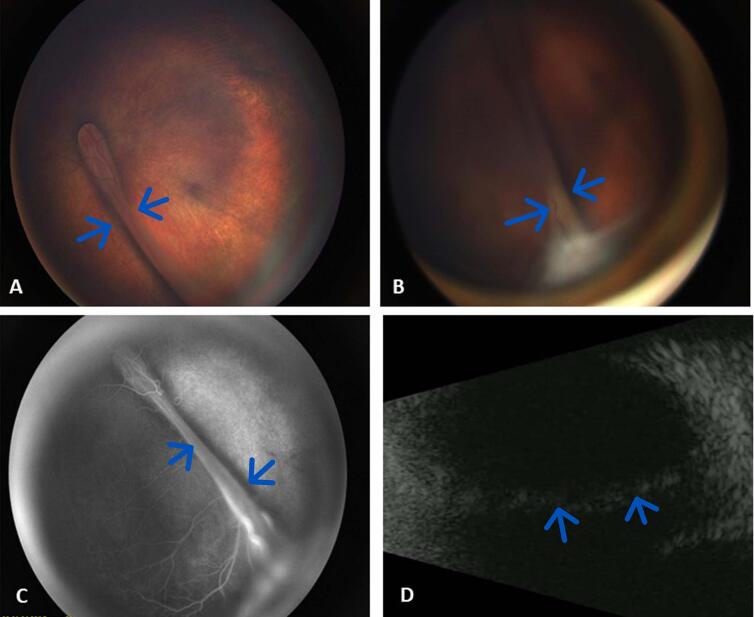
(Left eye). A. Retcam color fundus photo, focused posterior. B. Retcam color fundus photo, focused anterior showing knife fold retinal detachment with anterior fibrosis to posterior lens capsule. C. Fluorescein angiography, late image. D. B-Scan ultrasound confirming knife fold configuration retinal detachment emanating from the optic nerve and propagating towards the inferotemporal periphery.
